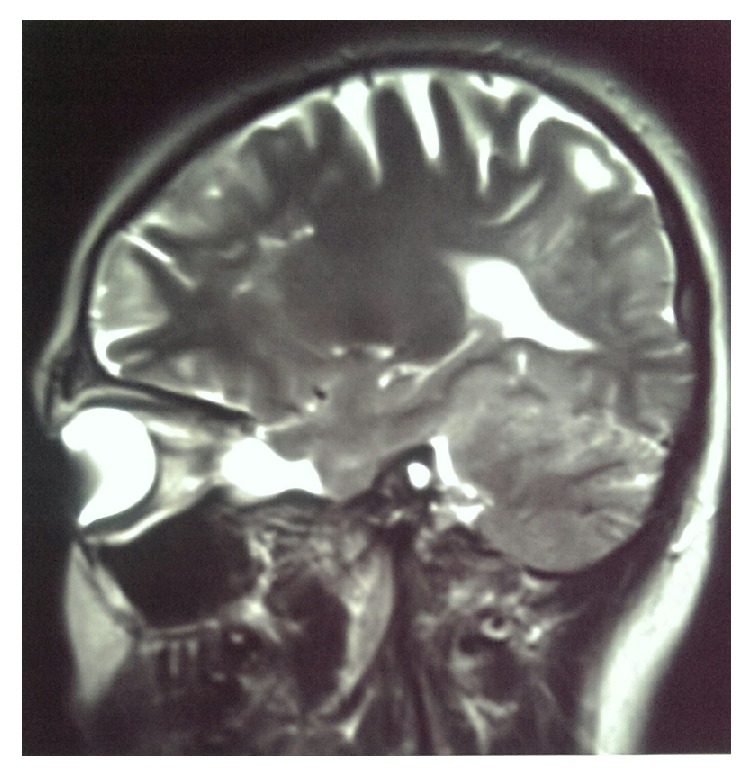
T2 weighted MRI of the brain in sagittal section, showing low-signal subependymal lesion beneath lateral ventricles along with bilateral supratentorial subcortical flair hyperintense signal lesions.
